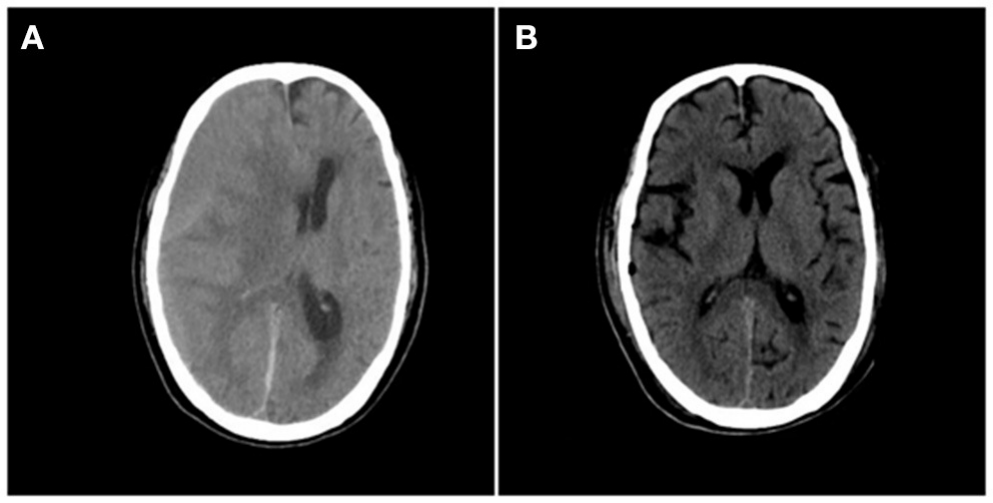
(A) Preoperative CT showing a crescent-shaped slightly dense shadow under the right cranial plate, with displaced midline structures and lateral ventricular compression. (B) Postoperative brain CT reexamination showing complete hematoma clearance without secondary bleeding.
